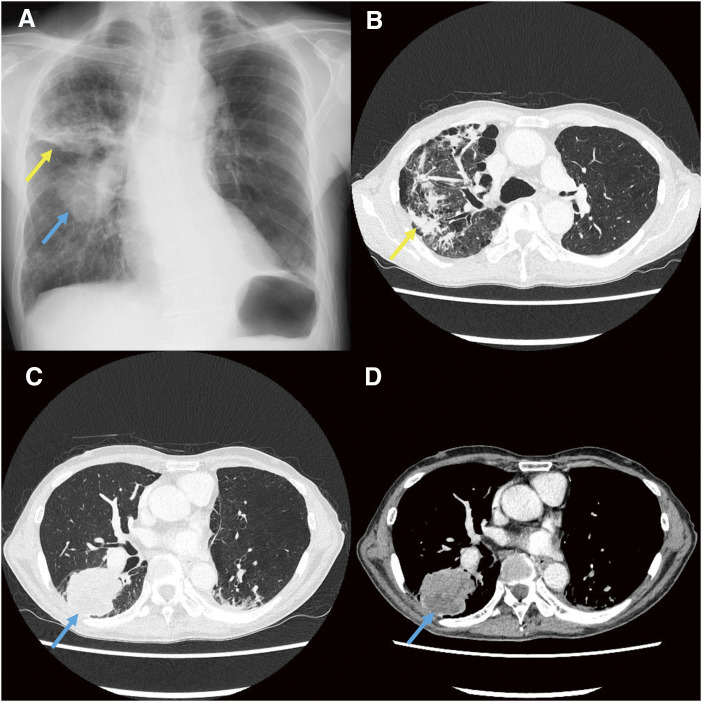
Chest radiograph and chest CT on admission. (A) Radiograph showing the independent existence of an infiltrate (yellow arrow) and a noticeable round mass (blue arrow) on the right hemithorax. (B) Contrast-enhanced CT (lung window setting) showing a patchy and irregular peripheral and peribronchial consolidation (yellow arrow) at the level of the upper lobes. (C) CT showing a 6-cm round tumor (blue arrow) at the level of the lower lobe. (D) CT (mediastinal window setting) showing a large low-density area (blue arrow) in the tumor. CT: computed tomography
